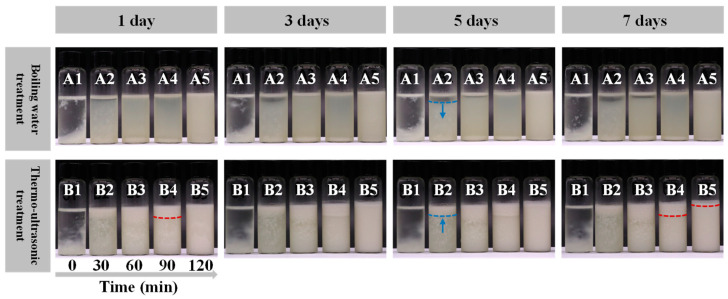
Appearance of the fish broth after storage at 1, 3, 5, 7 days by boiling water (A) and thermo-ultrasonic treatment (B) with different treatment times (1–5—0, 30, 60, 90, 120 min).
